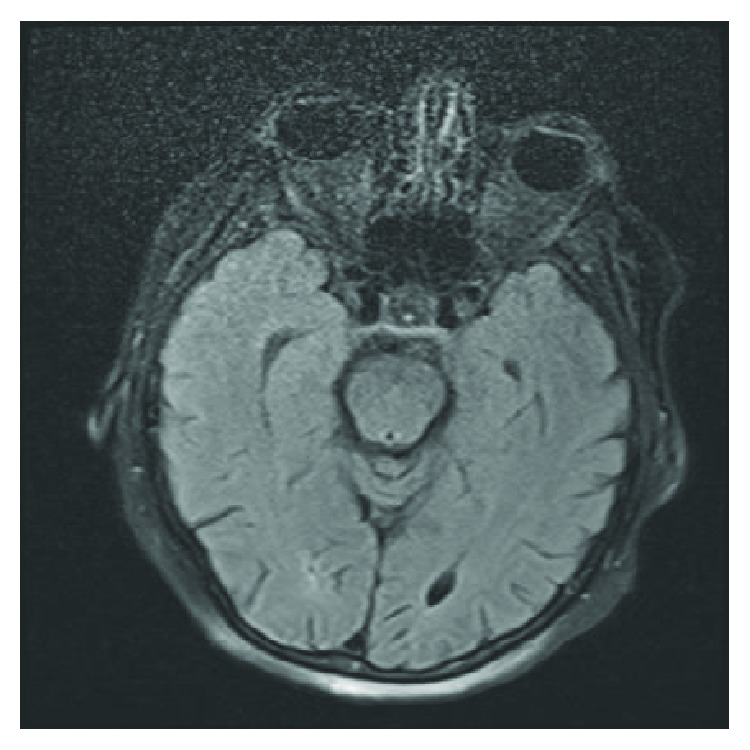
Axial T1-weighted sequence through the midbrain.
